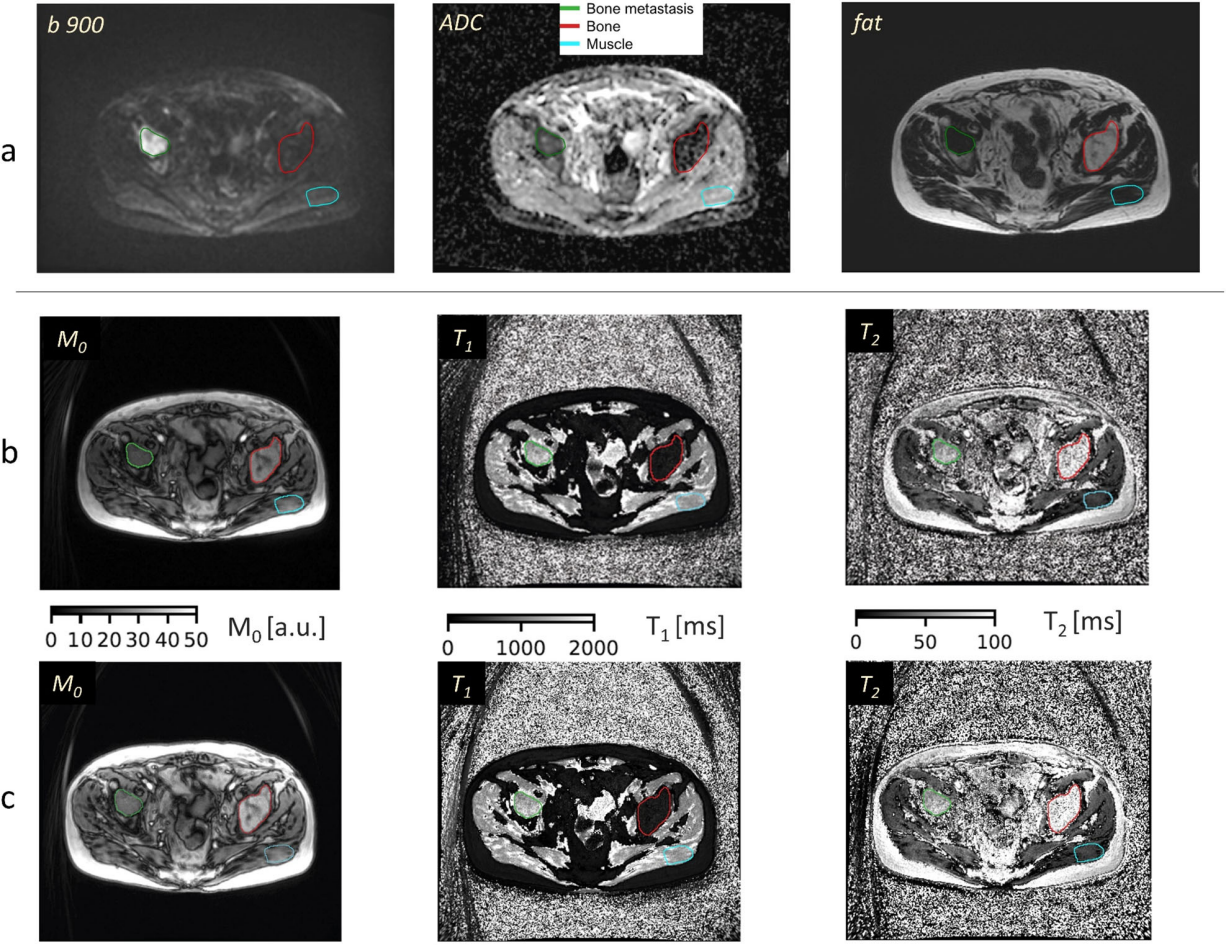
a Matched axial parametric maps derived from DWI and Dixon acquisitions for a 65-year-old patient, helping to differentiate between normal and metastatic bone. b, c Example of two repeated MRF measurements of M_0_, T_1_ and T_2_ derived from the same patient demonstrating an active bone metastasis in the right iliac bone (green), normal-appearing bone (red) and gluteus muscle (blue). *ADC*, apparent diffusion coefficient
